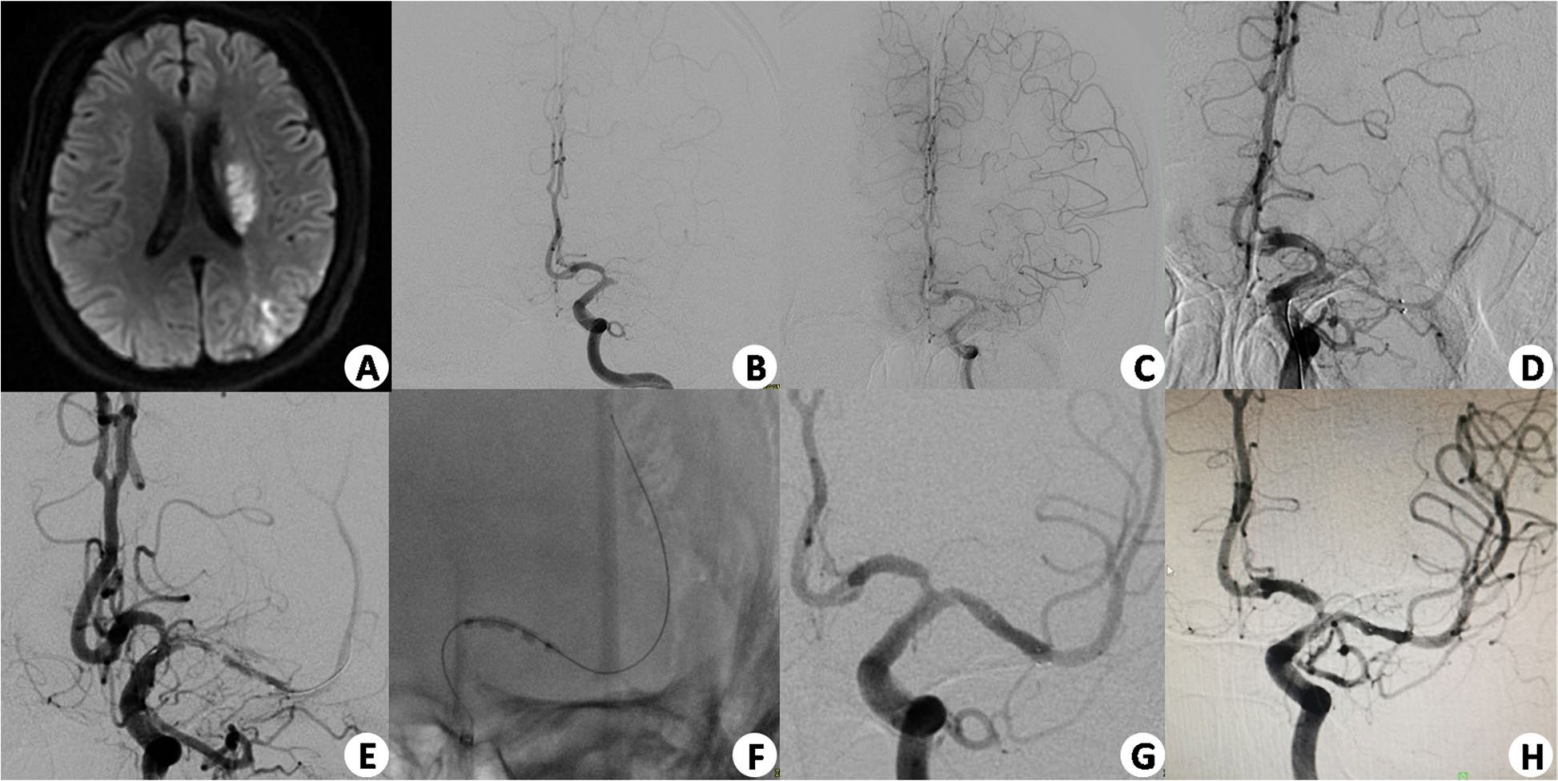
A 29-year-old male patient with acute ischemic stroke receiving bridging therapy, NIHSS 10. A Preoperative MRI showed an acute infarction in the left cerebral hemisphere. B, C, and D DSA showed left M1 occlusion with good collaterals, and this patient was treated with endovascular thrombectomy. E Repeat angiography showed an intracranial atherosclerotic severe stenosis in the lesion. F and G This patient received a rescue therapy including balloon angioplasty (Gateway 2.5 × 15 mm) with stenting (Neuroform 3.5 × 15 mm), and the outcome was mTICI 3. H A 6-month follow-up angiogram showed good stent apposition to the parent artery wall, and the degree of stenosis was worse compared with the initial outcomes after procedure
